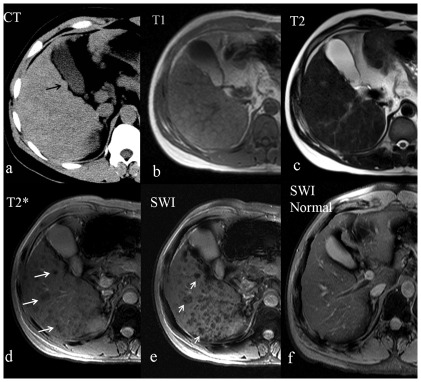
Representative images from a single patient comparing CT and multiple MR imaging methods. A 37 year-old man with hepatitis-B induced cirrhosis. (a) CT image demonstrates a high-attenuation siderotic nodule in the gallbladder fossa (black arrow). (b) Transverse T1-weighted gradient echo image and (c) Transverse T2-weighted fast spin echo image with a few hypointense nodules. (d) Transverse T2*-weighted GRE image demonstrated numerous hypointense nodules with different sizes (long arrows). (e) Transverse susceptibility weighted image (SWI) shows a greater number of hypointense lesions, with a higher contrast relative to background parenchyma (short arrows). (f) SWI comparison image from a normal 37-year-old man.
